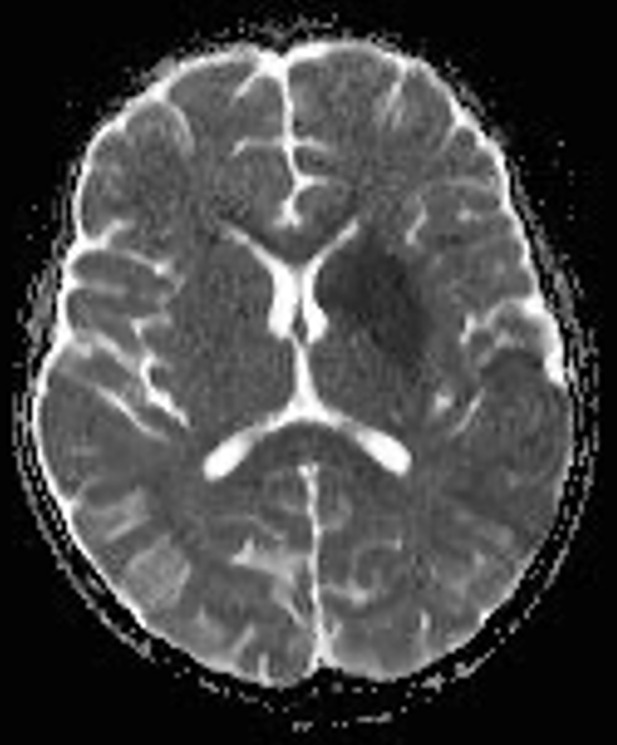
ADC axial slice of MRI brain showing areas of restriction on diffusion weighted images are evident involving caudate nucleus, putamen, insular cortex and parietal lobe
